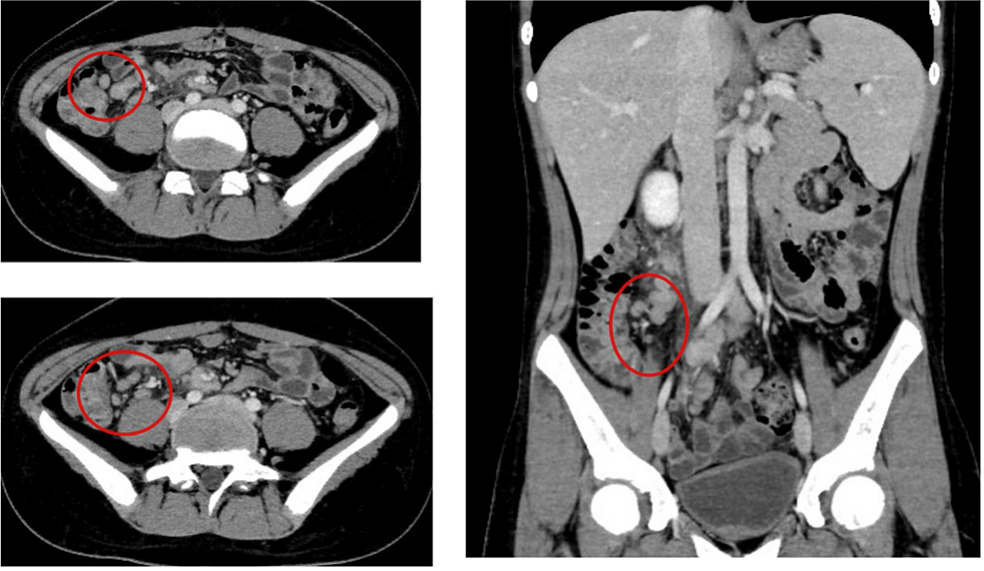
Contrast-enhanced computed tomography depicting enlarged lymph nodes around the ileum and ileocecal region (red circles).
